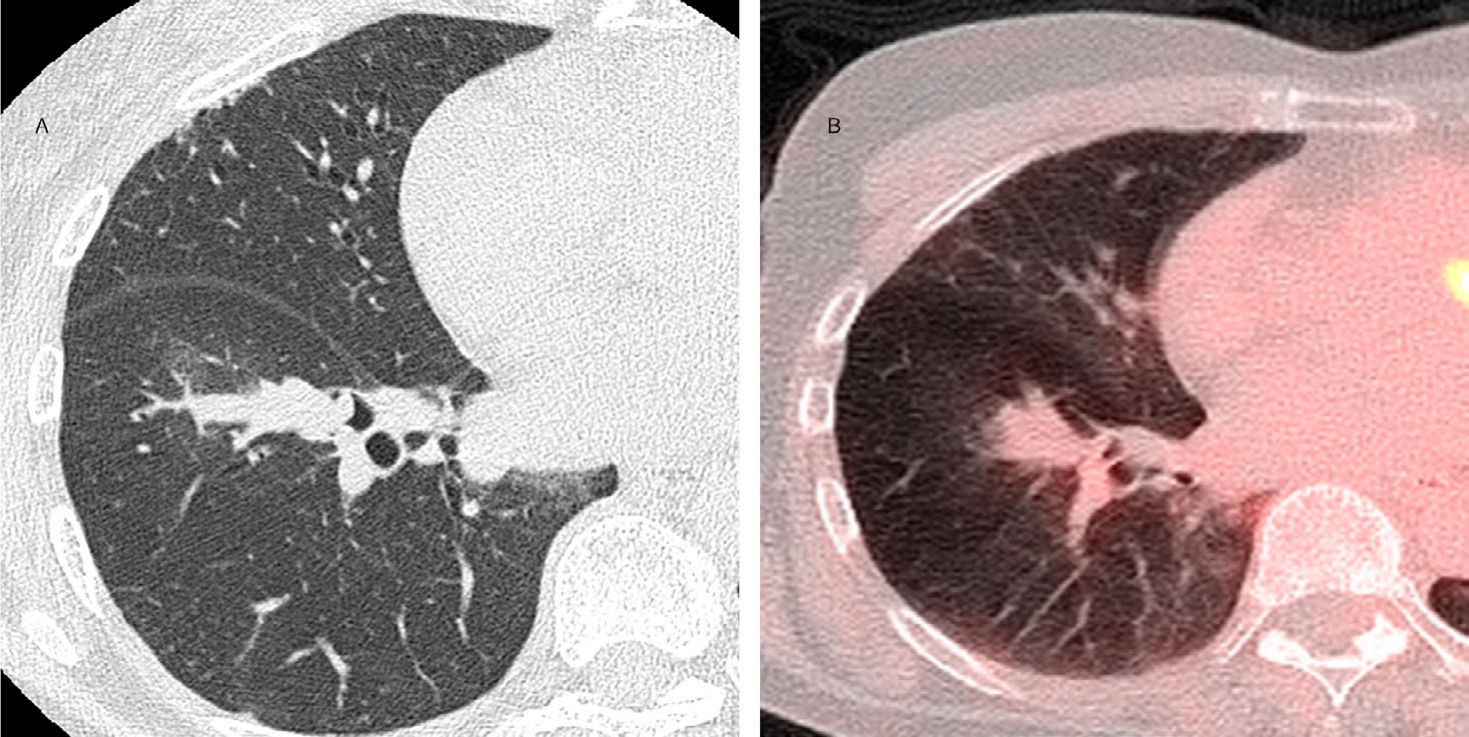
Chest imaging at the initial assessment. Computed tomography (CT) of the chest demonstrated a well‐defined 30‐mm mass in the B8/9 segment of the right lower lobe, extending into the bronchial lumen. The lesion exhibited no evidence of cavitation or necrosis. There were no signs of regional lymphadenopathy or distant metastasis. Fluorodeoxyglucose positron emission tomography‐computed tomography (FDG/PET‐CT) revealed an increased FDG uptake in the mass (B) with a maximum standardized uptake value (SUV) of 1.77. No other FDG uptake was observed.
